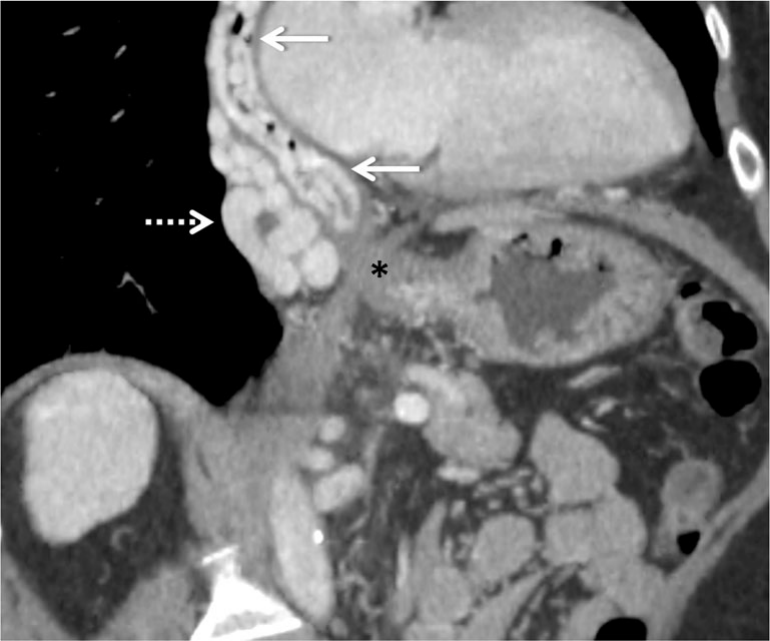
Coronal-oblique maximum intensity projection (MIP) contrast-enhanced CT (CECT) image demonstrating multiple collaterals in the oesophageal mucosa (*solid arrows*) and para-oesophageal region (*interrupted arrow*). *Asterisk* denotes the gastro-oesophageal junction
